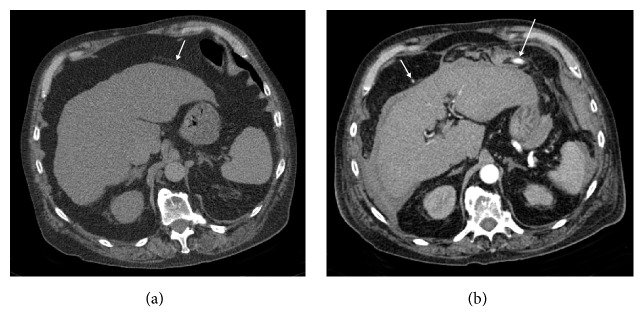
Computed tomography (CT) images. CT on admission (a) demonstrating liver cirrhosis with the greater omentum in the upper abdomen and tiny omental vessels (arrow) in the left upper abdomen. Arterial phase of contrast-enhanced computed tomography image six days later (b) with haemoperitoneum, segmental dilatated omental artery in the left (arrow), and spot of enhanced tiny omental artery in the right upper abdomen (small arrow).
